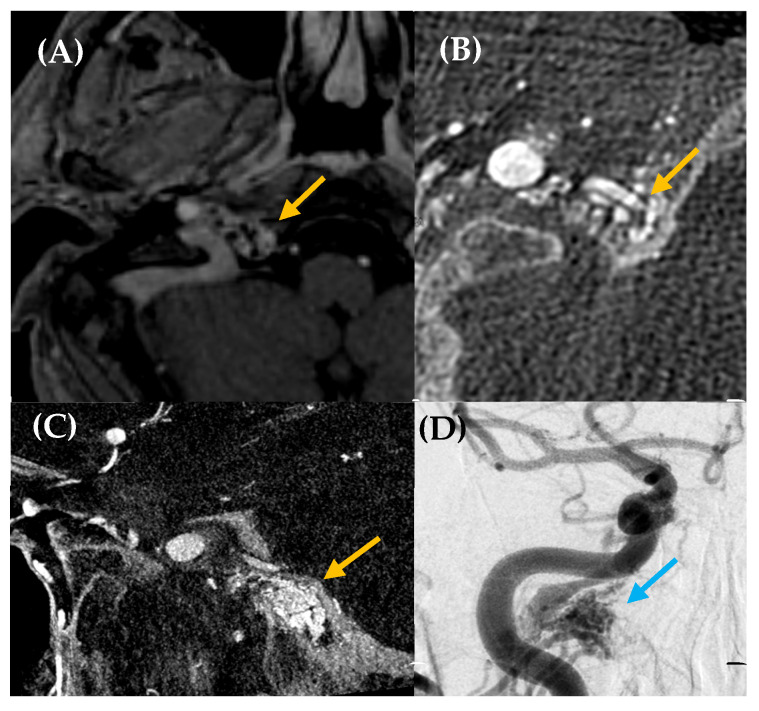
High-flow arteriovenous shunt located in the right hypoglossal canal. A 59-year-old man with a history of multiple cardiovascular risk factors and cardiac conditions presented with a somatosound in the right ear for several years. Otoscopy was unremarkable. Axial post contrast T1-weighted 3D MRI (image (A)) was performed, revealing a likely dural arteriovenous fistula (dAVF) in the petrous, with predominantly posteroinferior drainage (yellow arrow). Cone-beam CT angiography axial plane (images (B,C)) and DSA (image (D)) confirmed a high-flow arteriovenous shunt at the skull base, located in the right hypoglossal canal, measuring 15 × 16 mm (yellow arrow, images (B,C); blue arrow, image (D)). The fistula was primarily fed by the meningeal branch of the right occipital artery, with additional supply from neuromeningeal branches of the bilateral ascending pharyngeal arteries. Venous drainage occurred via the right inferior petrosal sinus with retrograde reflux into the cavernous sinus and the right superior ophthalmic vein, without extension into the intracranial pial veins. Embolization was performed as treatment, leading to the resolution of the patient’s PT.
